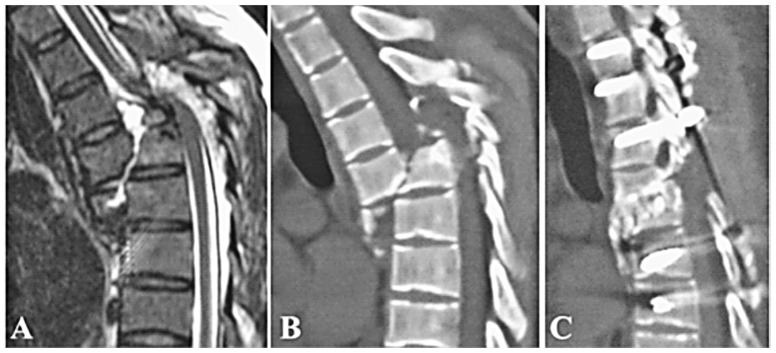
Type B1.2.3 severe fracture-dislocation involving T5-6; the disc was squeezed out of disc space posteriorly (black arrow) and anteriorly (white arrow) and intervened between two fractured vertebrae (A,B). Posterior reduction, instrumentation with 9 pedicle-screws, decompression, T5-6 disc excision, and bone graft in disc space were performed at one stage. One year later, follow-up CT showed good alignment and bony fusion (C).
